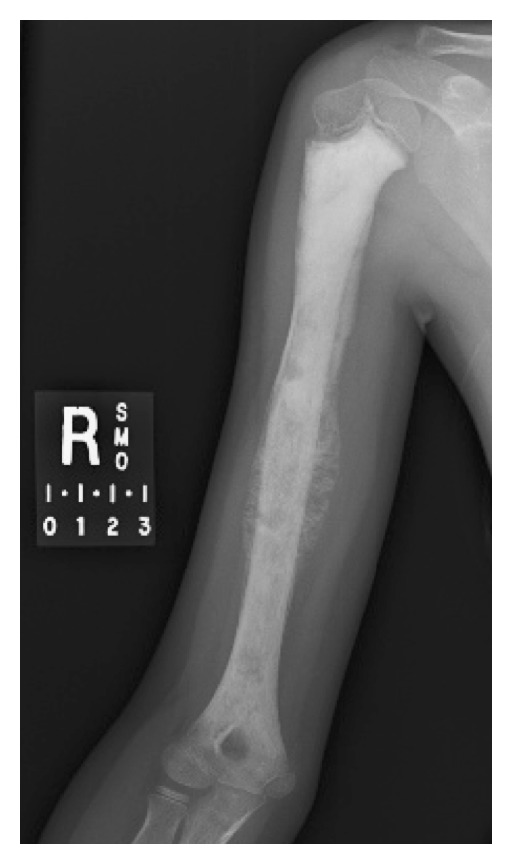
Anteroposterior radiograph of humerus following neoadjuvant chemotherapy for osteosarcoma.
